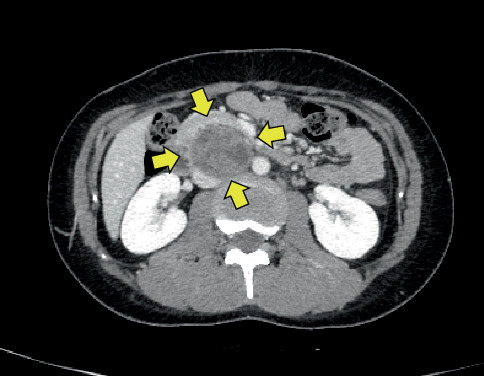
Computed tomography image showing enlarged para-aortic lymph nodes, the largest measuring 5.5 cm in diameter.
